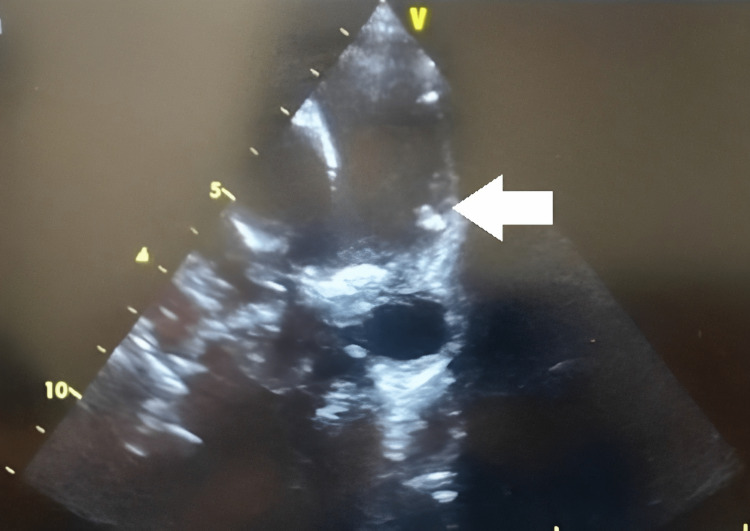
The transthoracic echocardiology (TTE) showed vegetation (white arrow) attached to the pulmonary artery
